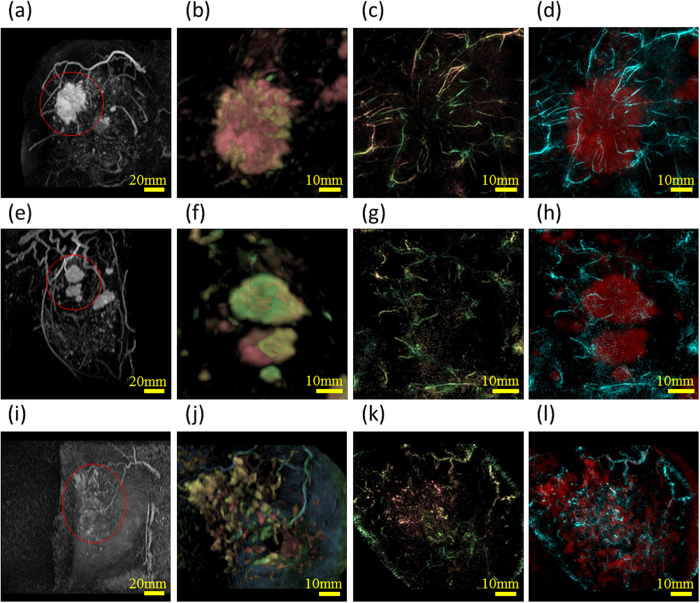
Examples of peritumoral images from three cases. Figures of the first column from the left are the original MR images. Each lesion is indicated by a red circle. Those of the second column are the enlarged MR images around the lesion after deforming into the shape to PA images. The third and the fourth are original PA images, and fusion images of PA (cyan) and MR (red), respectively. These figures are after eliminating the PA signals from subcutaneous blood vessels by a depth of 4 mm. Case 3 (a) –(d): A 40-year-old woman with IBC. The tumor is 47 mm in diameter. Tumor-related blood vessels seem to converge from the normal breast tissue toward the center of the tumor, becoming drastically narrower at the tumor edge and nearly vanishing near the center. Case 4 (e) – (h): A 44-year-old woman with multiple IBCs. Tumor-related blood vessels seem to converge toward the center of the tumor in several masses. Case 5 (i) – (l): A 46-year-old woman with DCIS in upper-outer area in right breast. Non-mass enhancement was seen by MRI almost in the center of the breast. Centripetal blood vessels are hardly evident around the tumor. Spotty signals are seen in the PAI.
